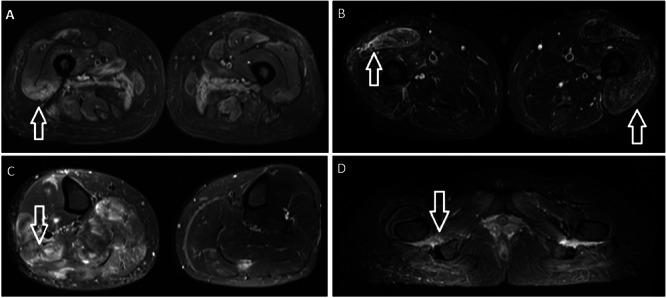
Example MRI scans showing myositis in patients with anti‐SRP antibody. (A) MRI thighs T2 image. Bilateral patchy areas of muscle oedema involving the abductor, adductor, extensor and hamstring muscles of the pelvis and both thighs. (B) Relatively symmetric oedema along the muscles in the anterior and posterior compartments of the thigh with relative sparing of the muscles in the posterior compartment. (C) Muscle oedema in both calves, more prominent on the right, without any atrophy/fatty infiltration. (D) Marked oedema in association with the quadratus femoris muscles.
